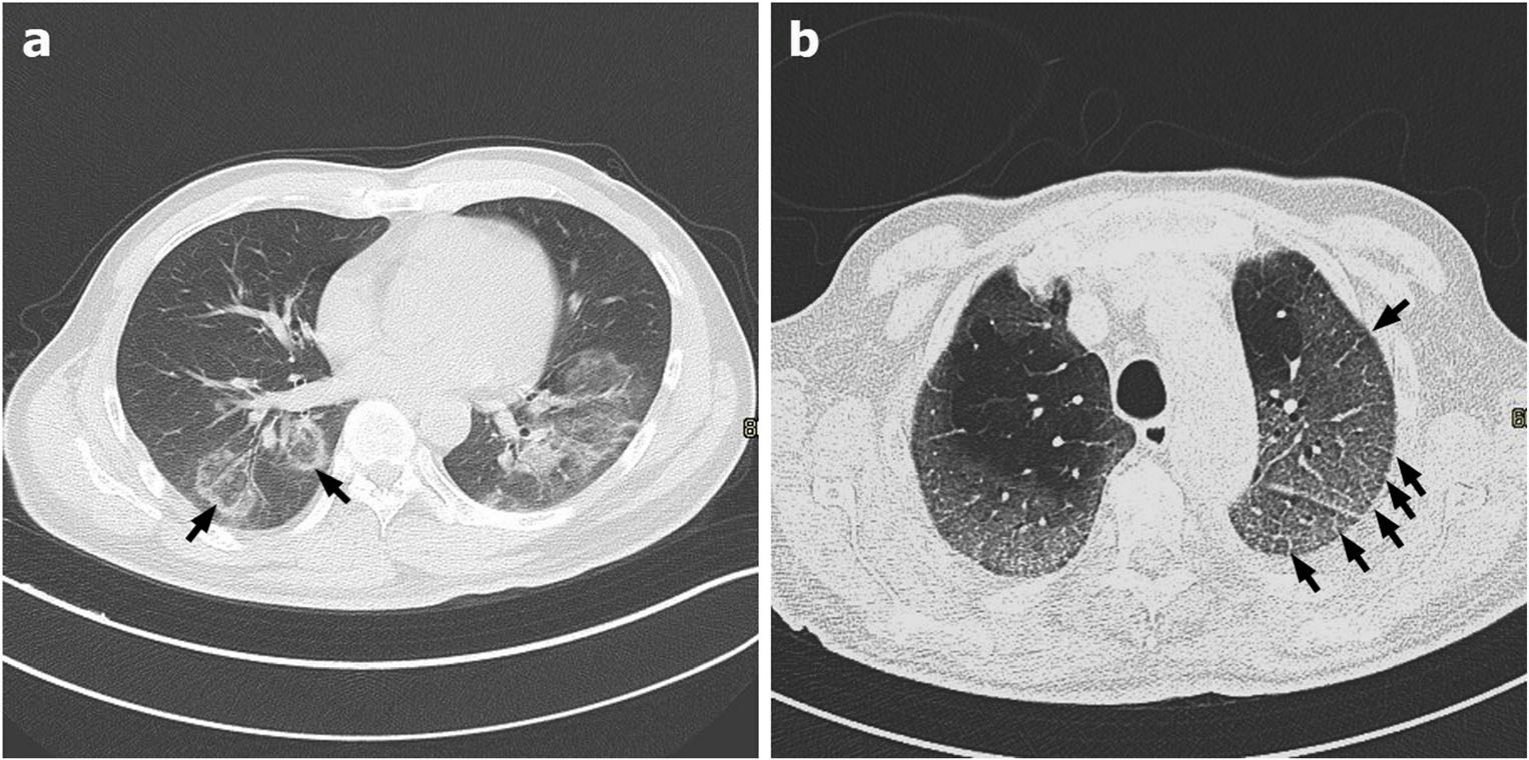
a–b Axial HRCT images of two patients with COVID-19 pneumonia showing an organizing pneumonia pattern (a), with bilateral ground-glass opacities, lower lobes prevalent distribution, and the classic reverse halo sign, or atoll sign (black arrows in a), in the right lower lobe; and a “crazy paving” pattern (b) resulting from ground-glass opacities superimposed to interlobular septal thickening (black arrows in b)
